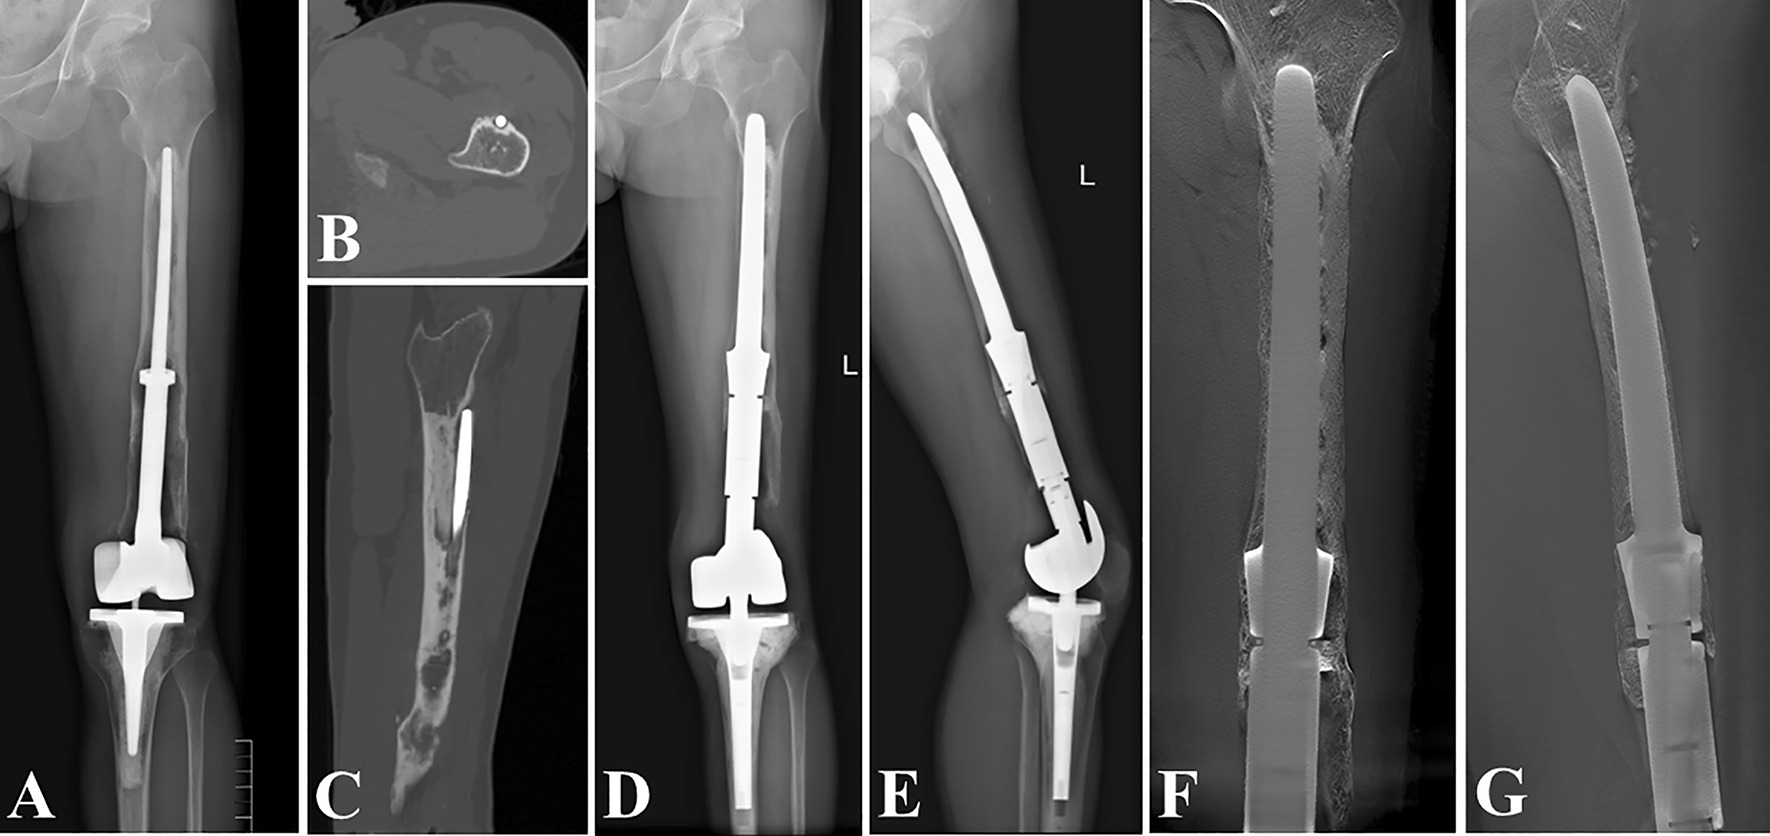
Revision of a Grade II bone loss case with “Long uncemented stem”. A–C preoperative radiographs; the anteroposterior and lateral radiographs (D, E), and the digital tomosynthesis images (F, G) 3 years after revision surgery
